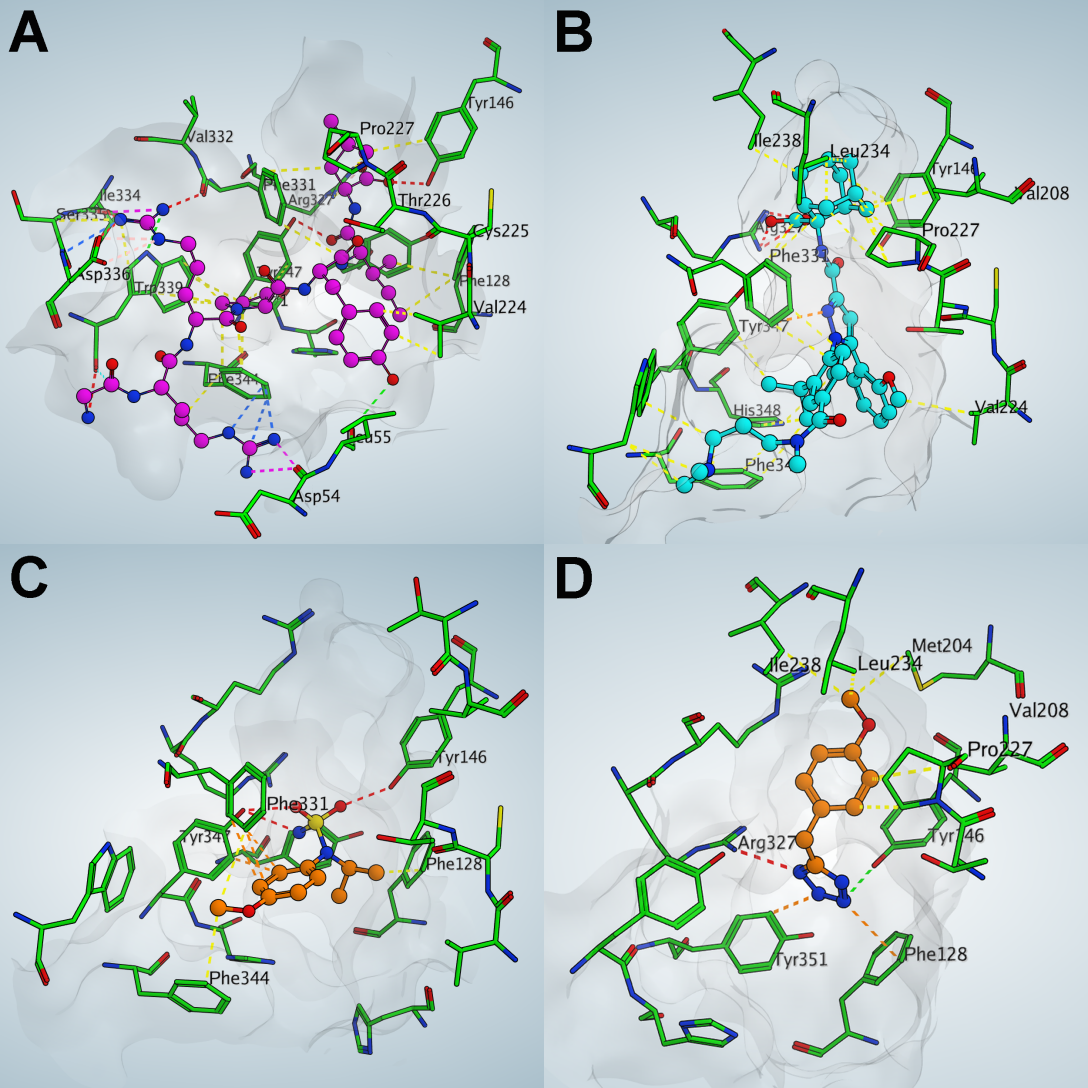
Comparison of the experimental X-ray structure complex of the peptidic agonist (NT)_8-13_ with the antagonist SR142948 as well as fragment structures as derived from docking experiments. (A) The binding mode of the peptidic agonist (X-ray structure [4] shown as ball and stick, with carbon atoms colored in pink) in NTS1–H4 (shown as stick, with carbon atoms colored in green) binding pocket (shown as the molecular surface colored as white). Nitrogen atoms are colored in blue, and oxygen atoms are colored in red in both, ligand and protein. Yellow, red and orange dash lines represent hydrophobic, electrostatic, and π-π interactions, respectively. The same rules are applied for the following figures. (B) The binding mode of the SR142948 antagonist (docked conformation shown as ball and stick, with carbon atoms colored in cyan) in NTS1 binding pocket.(C) The binding mode of fragment hit 2 (docked conformation shown as ball and stick, with carbon atoms colored in orange) in NTS1 binding pocket. (D) The binding mode of fragment hit 4 (docked conformation shown as ball and stick, with carbon atoms colored in orange) in NTS1 binding pocket.
